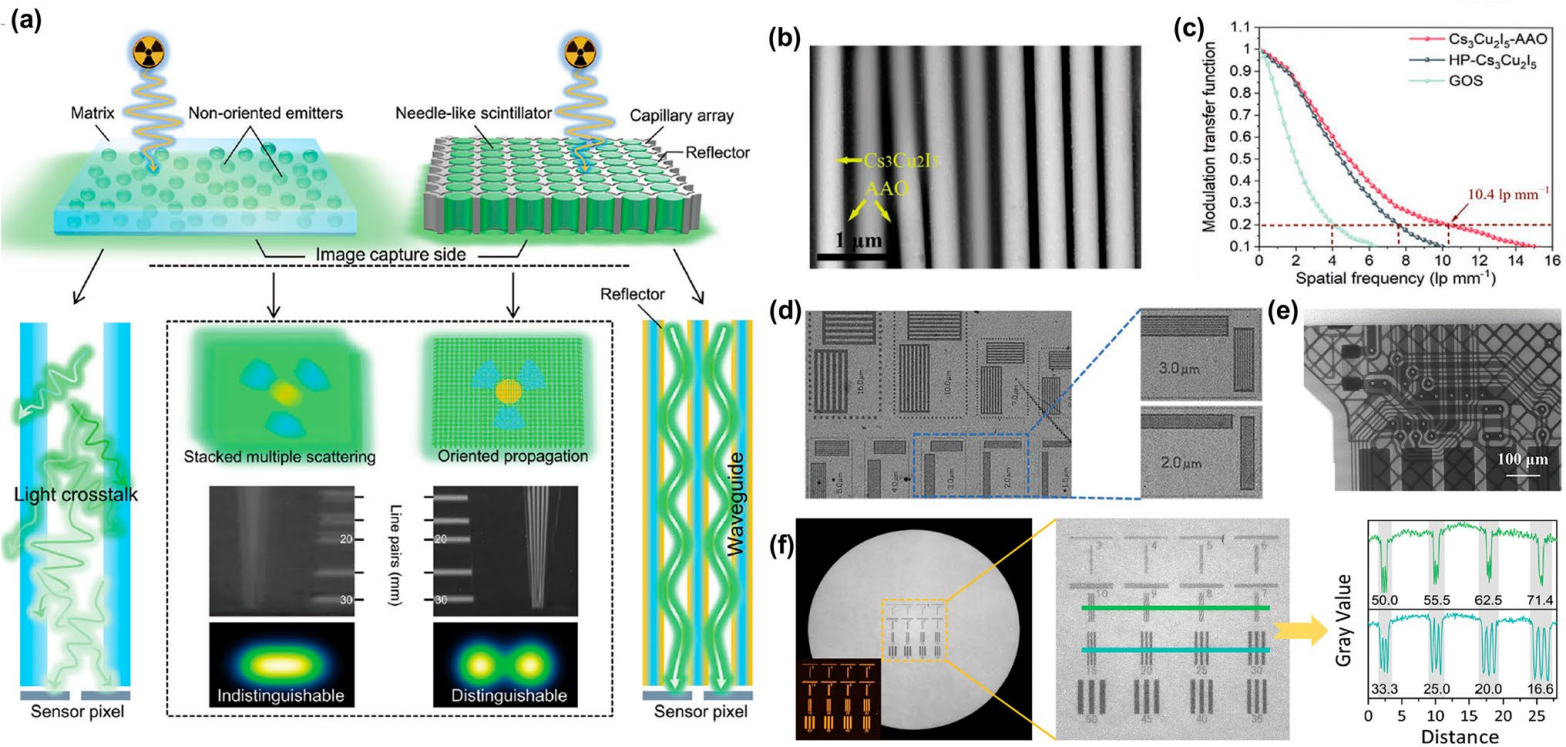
Scintillator performances of waveguided MHPs. a Light propagation mechanisms and results of conventional nonstructured scintillators and structured capillary needle-like array scintillators in X-ray imaging [154]. b Cross-sectional SEM image of Cs_3_Cu_2_I_5_-AAO scintillator. c The MTF values of Cs_3_Cu_2_I_5_-AAO, HP-Cs_3_Cu_2_I_5_, and GOS [155]. d Spatial resolution of the pixelated CsPbBr_3_-AAO arrays scintillation. e X-ray imaging of memory card with the pixelated CsPbBr_3_-AAO arrays [156]. f X-ray image of a microresolution chart. The right one is the gray value profiles along the cyan and green lines extracted from the X-ray image of the microresolution chart [154]
